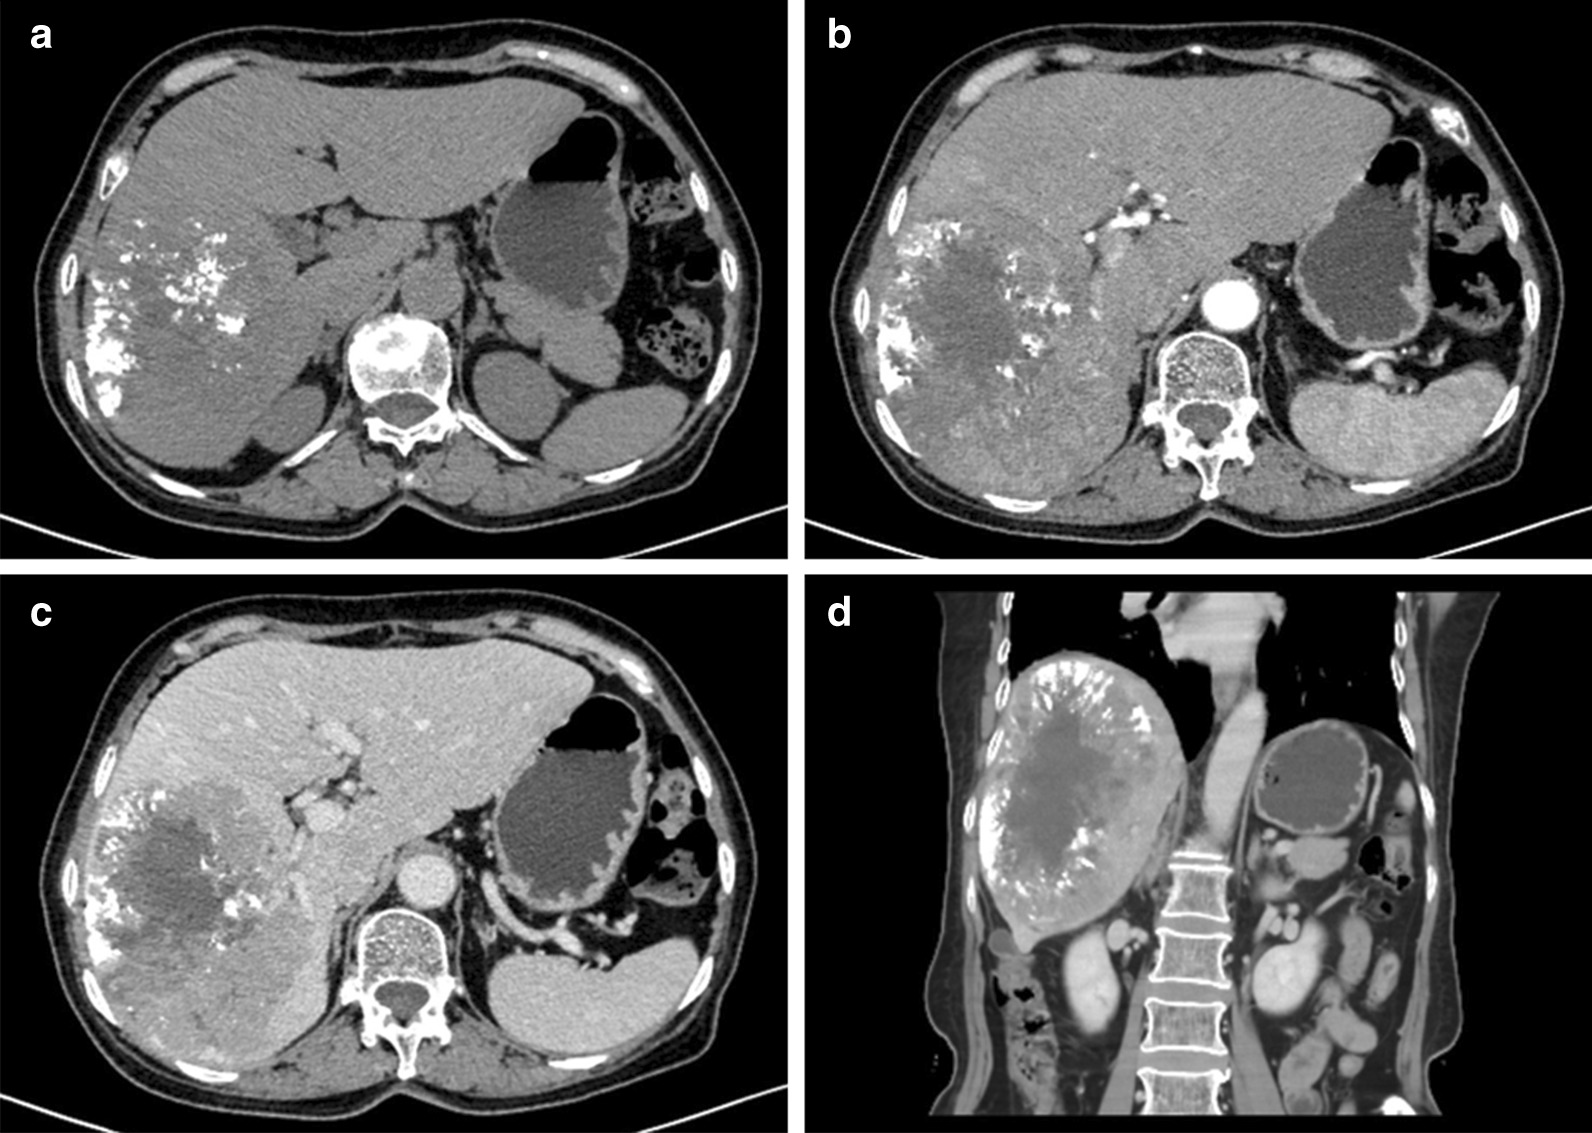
Abdominal CT images after TACE to observe the hepatoma. a Plain scan, b arterial phase, c venous phase, d coronal plane
